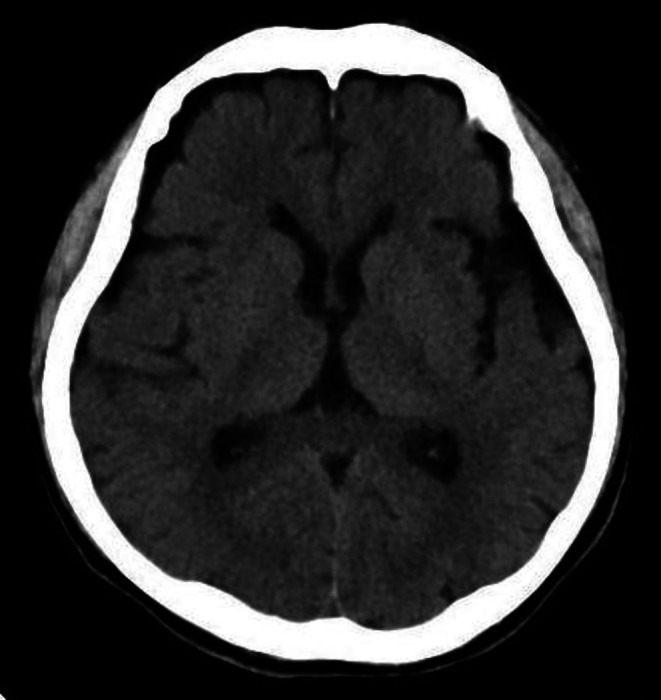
Computed tomography. No obvious pathological findings are observed.
